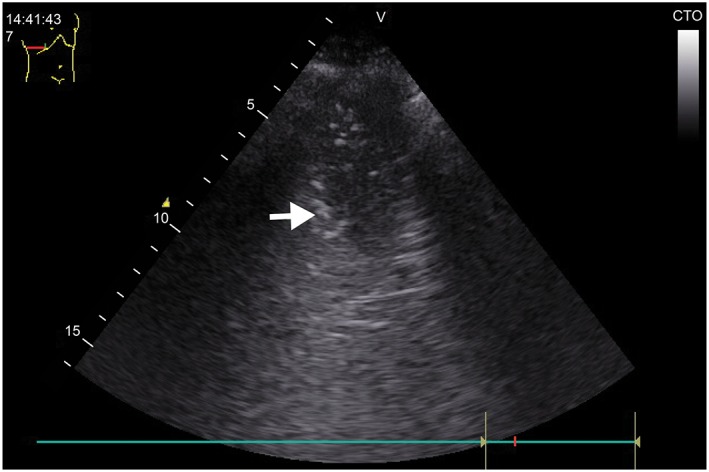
Ultrasound image. Alveolar consolidation with a frank tissue pattern arising from the pleural line with an irregular, shredded border (arrows). The shred sign is seen because the consolidation is in contact with the aerated lung
